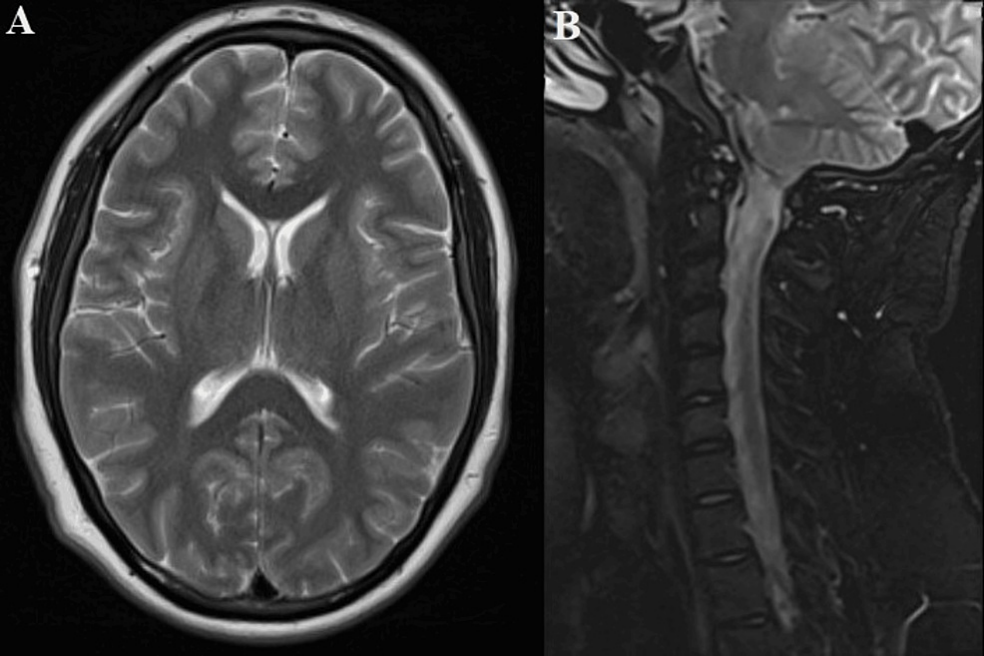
(A and B). MRI of the cranio-spinal transition. Figure 3A shows an axial section of the brain at the level of the lateral ventricles and thalamic structures, showing no abnormal imaging findings. Figure 3B shows a sagittal section of the cervical myelon where no MS plaques were detected.
